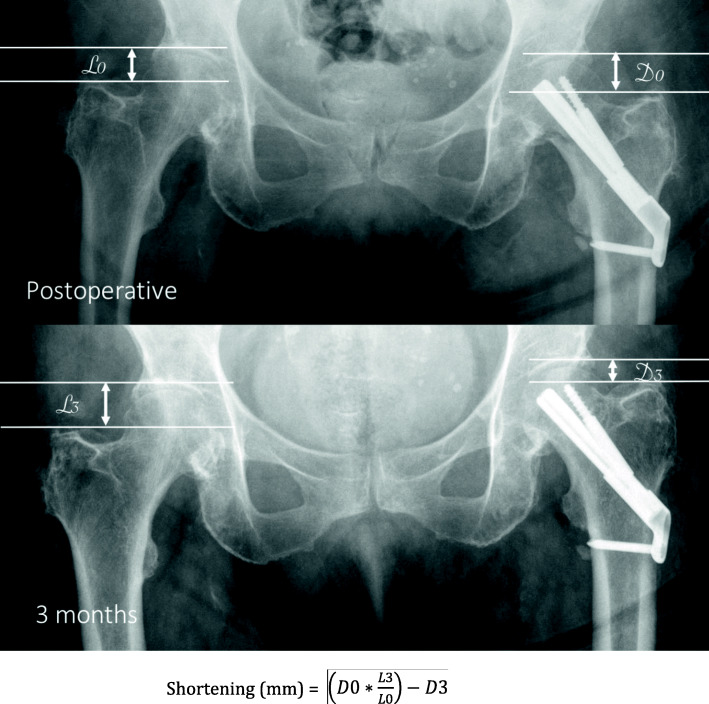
Calculation of proximal femur shortening with DHS, TS, and FNS. Postoperative and 3-month X-ray
